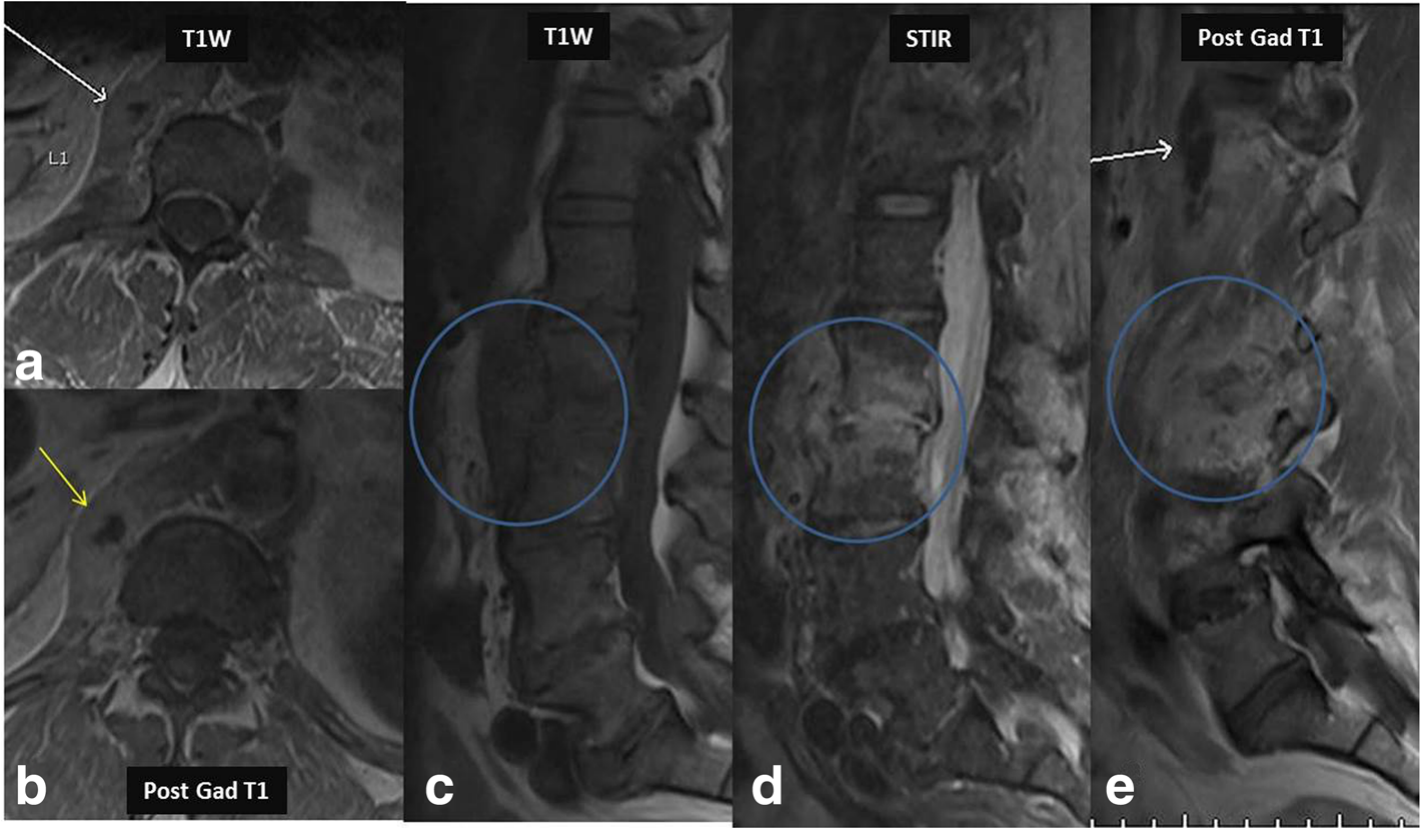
This is a companion case to the case shown in Fig. 2. 24-year-old male from India presented with low back pain. MR imaging showed involvement of the left diaphragmatic crura (*white arrow* in image a) and showing enhancement in post contrast imaging (*yellow arrow* in image b). Note the extensive prevertebral tubercular involvement (*blue circles* in c, d and e) on T1 W, STIR and post contrast imaging. *Staphylococcus aureus* was the causative organism
